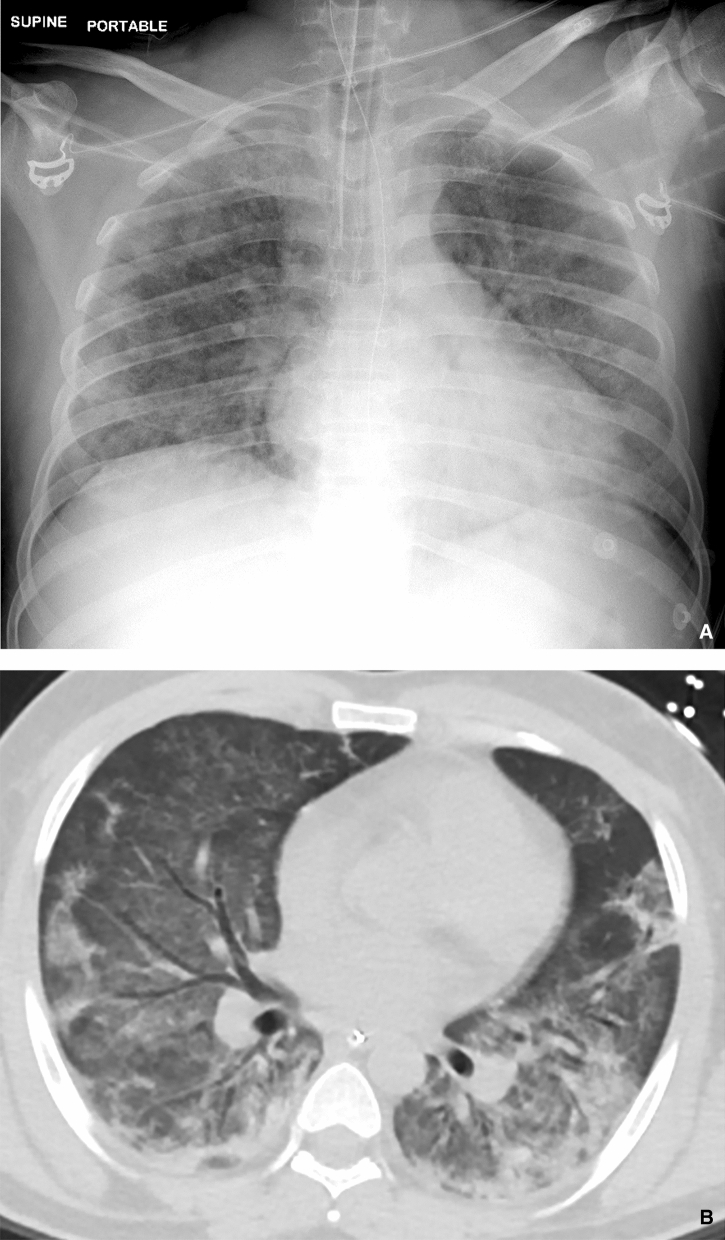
(a) Portable supine CXR shows intubated patient with diffuse mixed patchy ground glass opacities with consolidation predominantly in the peripheries. There is no zonal predilection, hilar adenopathy or associated pleural effusion. (b) Axial contrast enhanced CT (CECT) image across the lower zones of the lungs confirms CXR features. There are multifocal areas of consolidation in the peripheries, notably in the dependent regions of the lower lobes. Ground glass opacities are more diffusely distributed. Air bronchograms are salient throughout the lower lobes. There is notable absence of pleural effusions or adenopathy in the mediastinum (not shown).
